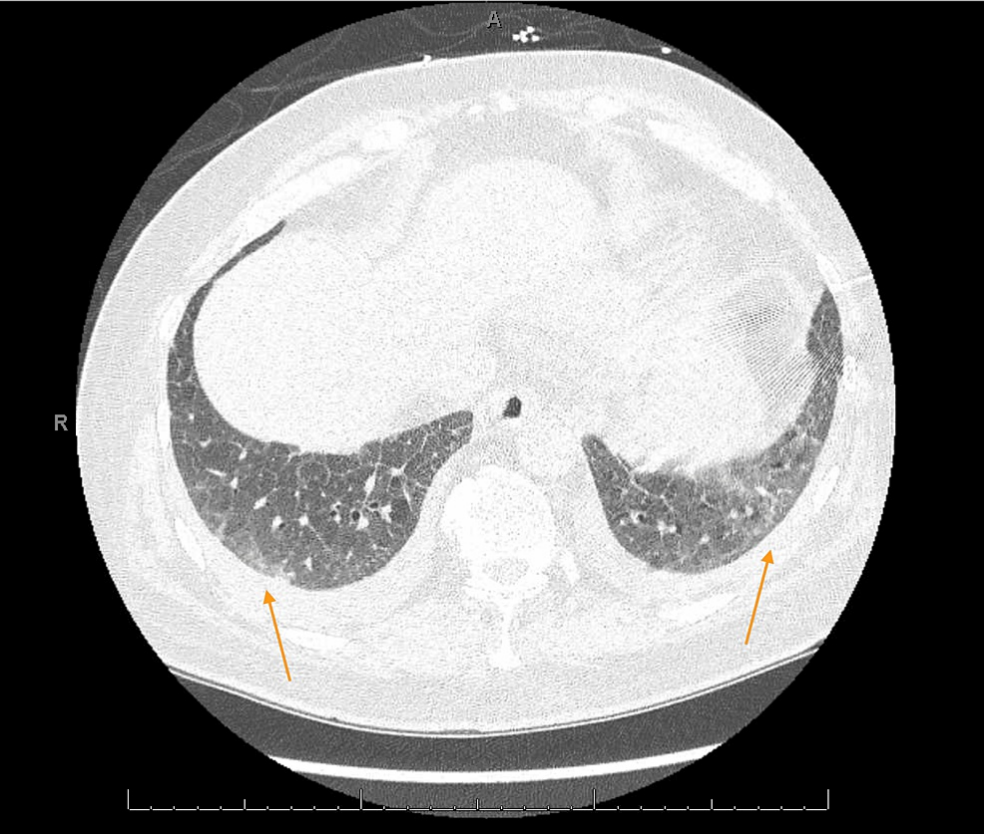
High Resolution CT Chest with Mild Bilateral Ground Glass Opacities, Middle Lobes
